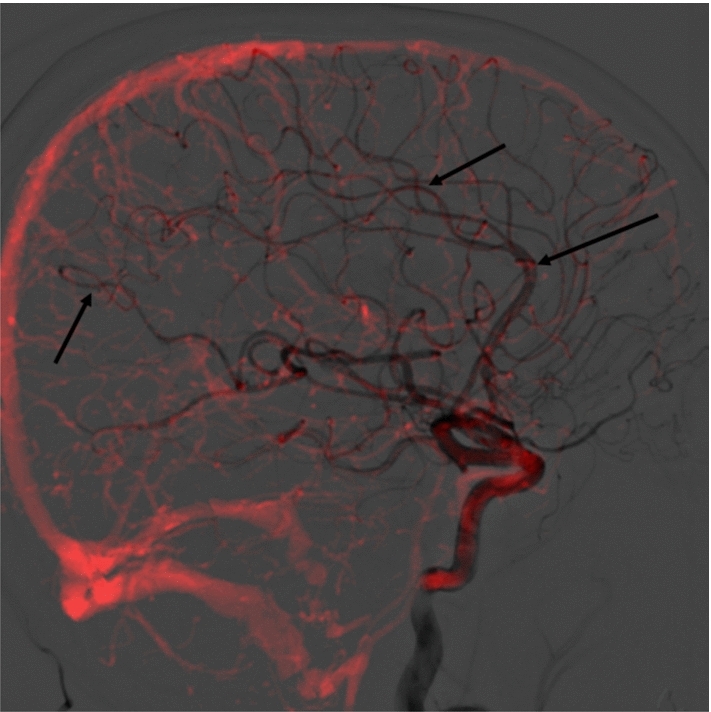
A CTA projected according to its registered pose over a DSA. CTA is shown with a red tint to enhance vessel visibility. Arrows point to locations containing smaller arteries that overlap in both the CTA and the DSA, which we hypothesize as being necessary for a finer-grained alignment
